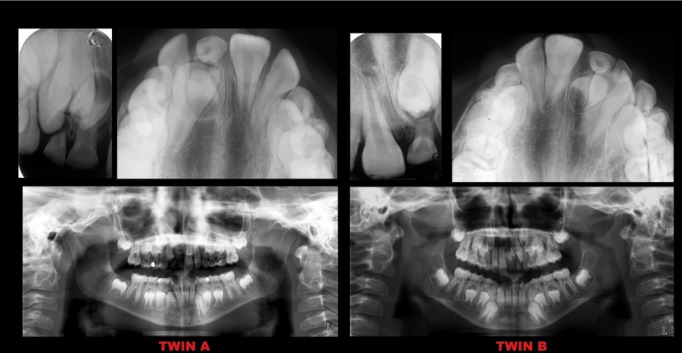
Panoramic x-ray, periapical and occlusal radiographs.
